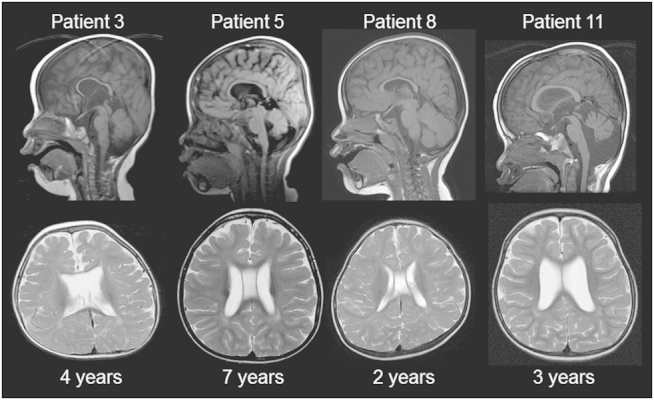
Brain magnetic resonance imaging (MRI) findings of new patients with Xq28 duplications. Sagittal T1 (up) and axial T2 (bottom)-weighted images are shown. All patients showed hypoplasia of the corpus callosum and T2 signal high intensities in the deep white matter. Three patients (other than patient 8) showed atrophies of the cerebellum and bilateral dilatations of the lateral ventricles, indicating age-dependent progression. Patient 3 showed a translucent septal defect, and patients 5 and 8 showed a verga cavity.
